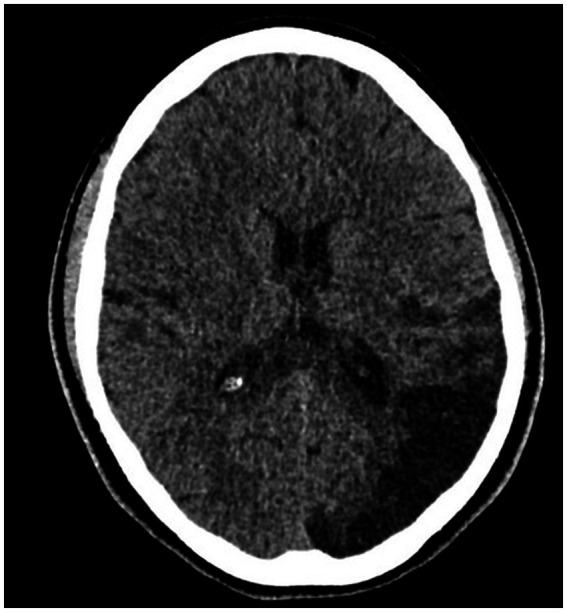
CT without contrast—ischemic changes in the left occipital lobe—the extent of vascularization of the terminal branches of the left posterior cerebral artery after the patient’s first stroke.
